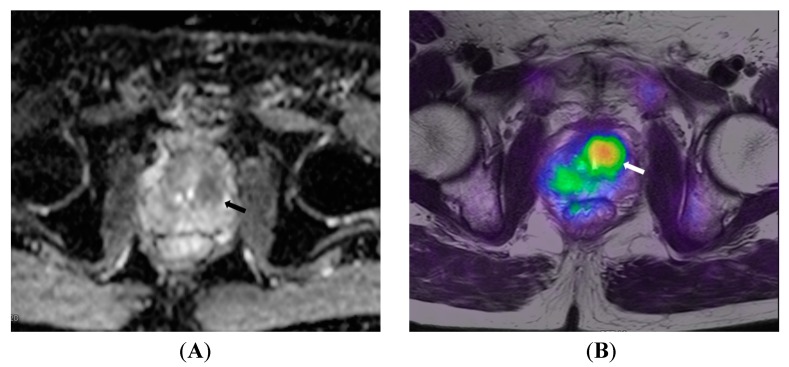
A 72-year old patient with prostate cancer. (A) ADC map shows low signal intensity in the central gland (arrow), which is confirmed by 18F-choline PET/MRI (arrow in B) to be metabolically active central gland tumor.
